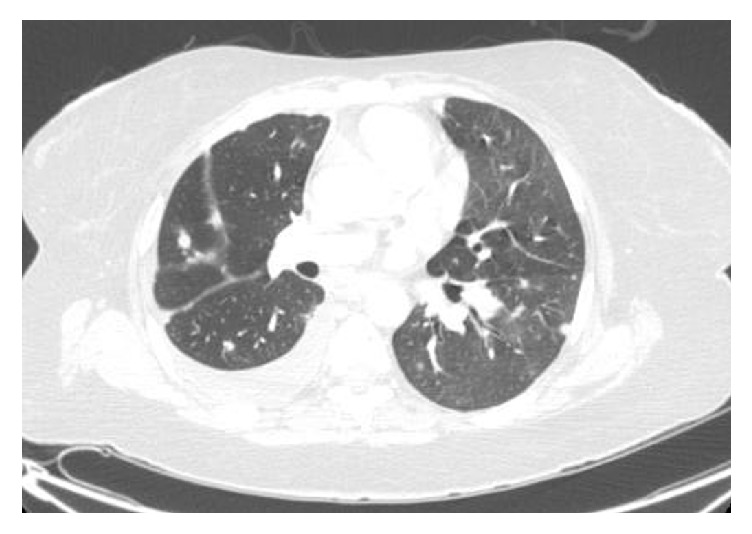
CT scan of chest showing a moderate right-sided pleural effusion, as well as bilateral pulmonary nodules with hilar adenopathy.
